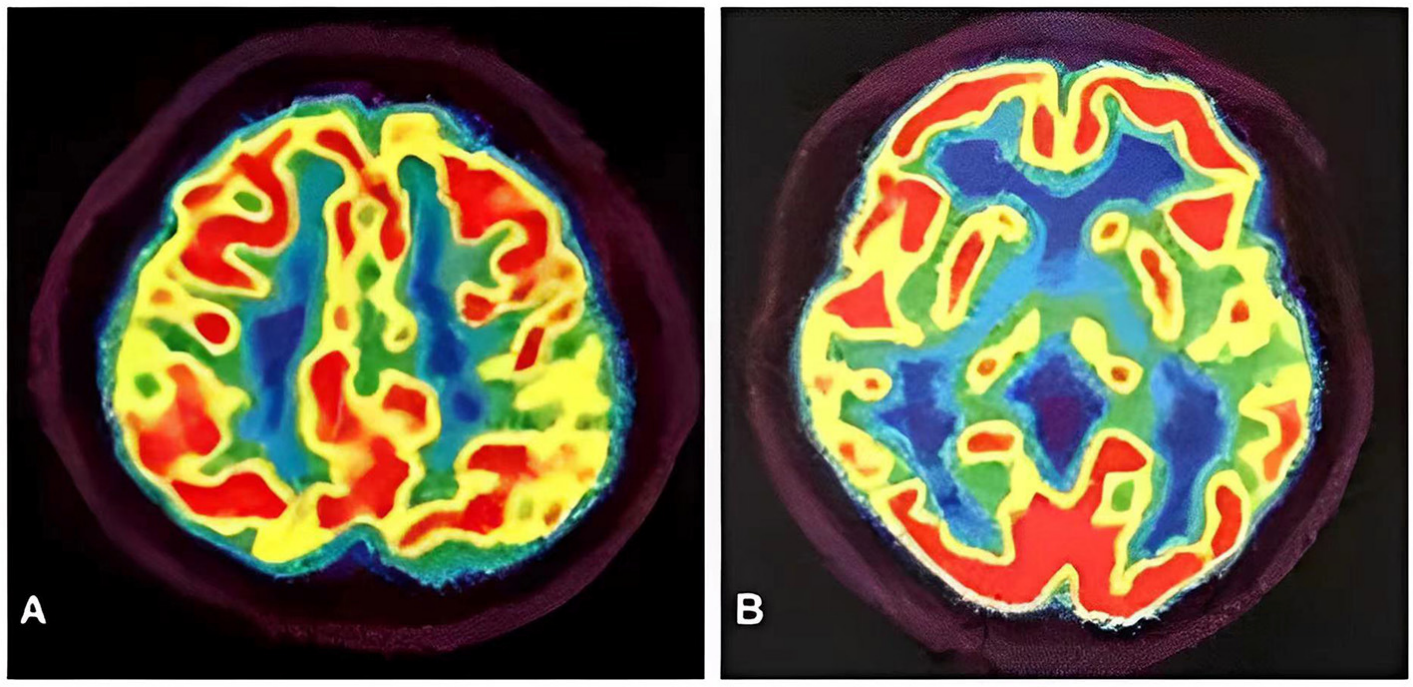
PET-CT images showed the left superior parietal lobe (A) and left middle temporal lobe (B) had mild to moderate decreased glucose metabolism, with reductions of 10 and 19%, respectively.
